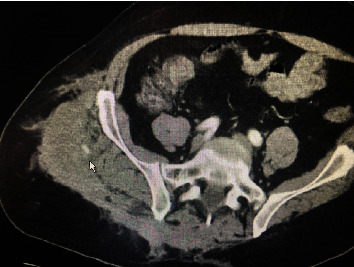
CT scan of the abdomen and pelvis for the first patient; the white arrow points towards a site of active extravasation (“blush”).
